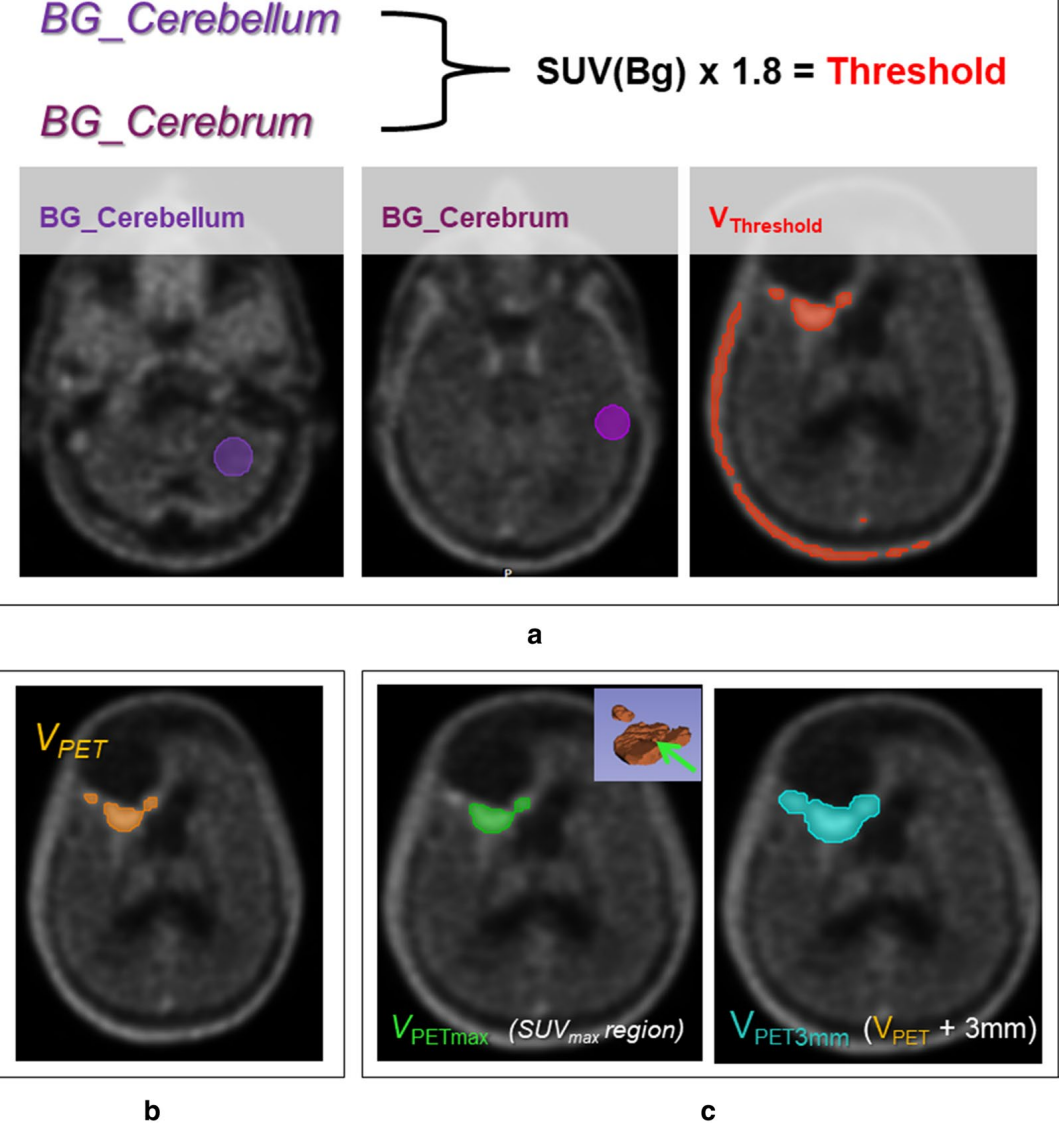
FET-PET segmentation steps: a for each patient a segmentation (V_Threshold_) was performed by a threshold of 1.8 times the FET uptake in background SUV(Bg.), with background defined by two spheres placed manually in cerebrum and cerebellum; b a radiation oncologist expert removed the regions which were not tumor-relevant and c two segmentations were defined on the remaining volume: V_PET3mm_ resulted from applying a margin of 3 mm around the whole PET volume (V_PET_) and V_PETmax_ was defined as the contiguous volume containing SUV_max_
